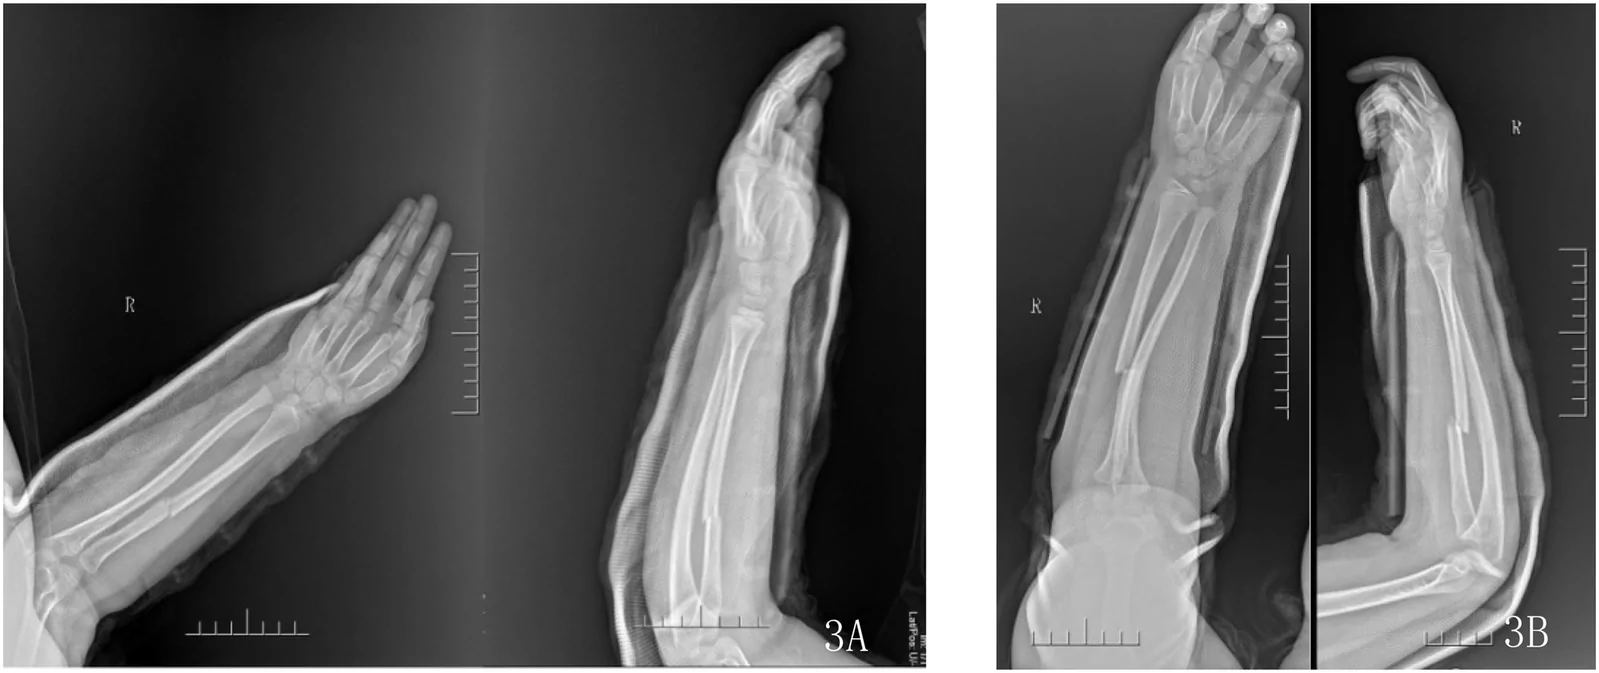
Post-manipulative reduction radiograph after refracture (A); seven-day follow-up outpatient radiograph demonstrating secondary fracture displacement (B).
